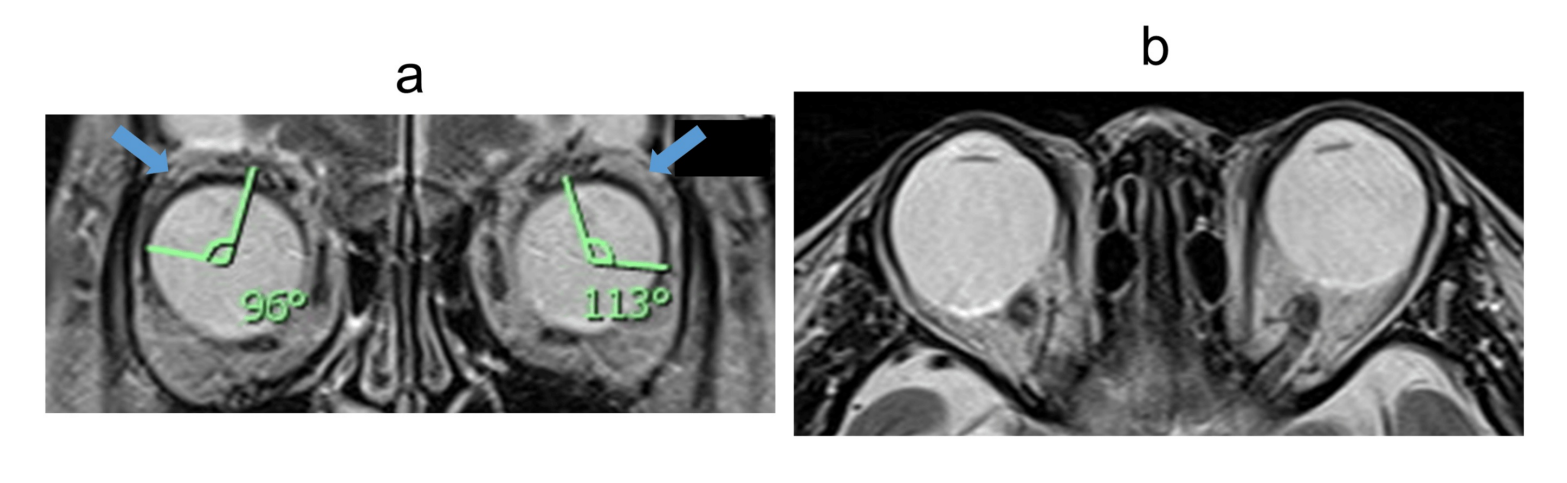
Orbital MRI of Case 2 (a) The angle formed by the centroids of the globe, superior rectus, and lateral rectus was slightly increased (right: 96°, left: 113°). The lateral rectus–superior rectus (LRSR) band was poorly visualized (blue arrow). (b) The globe appeared relatively large in relation to the orbital volume.
